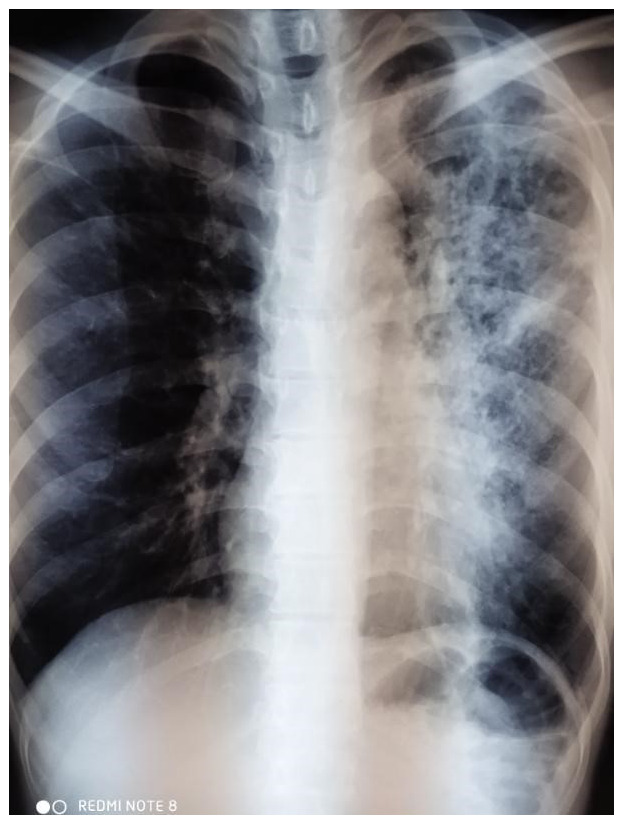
Chest X-ray showing thick walled cavitating lesions in the left upper lobe and patchy infiltrates in left middle and lower zones.
